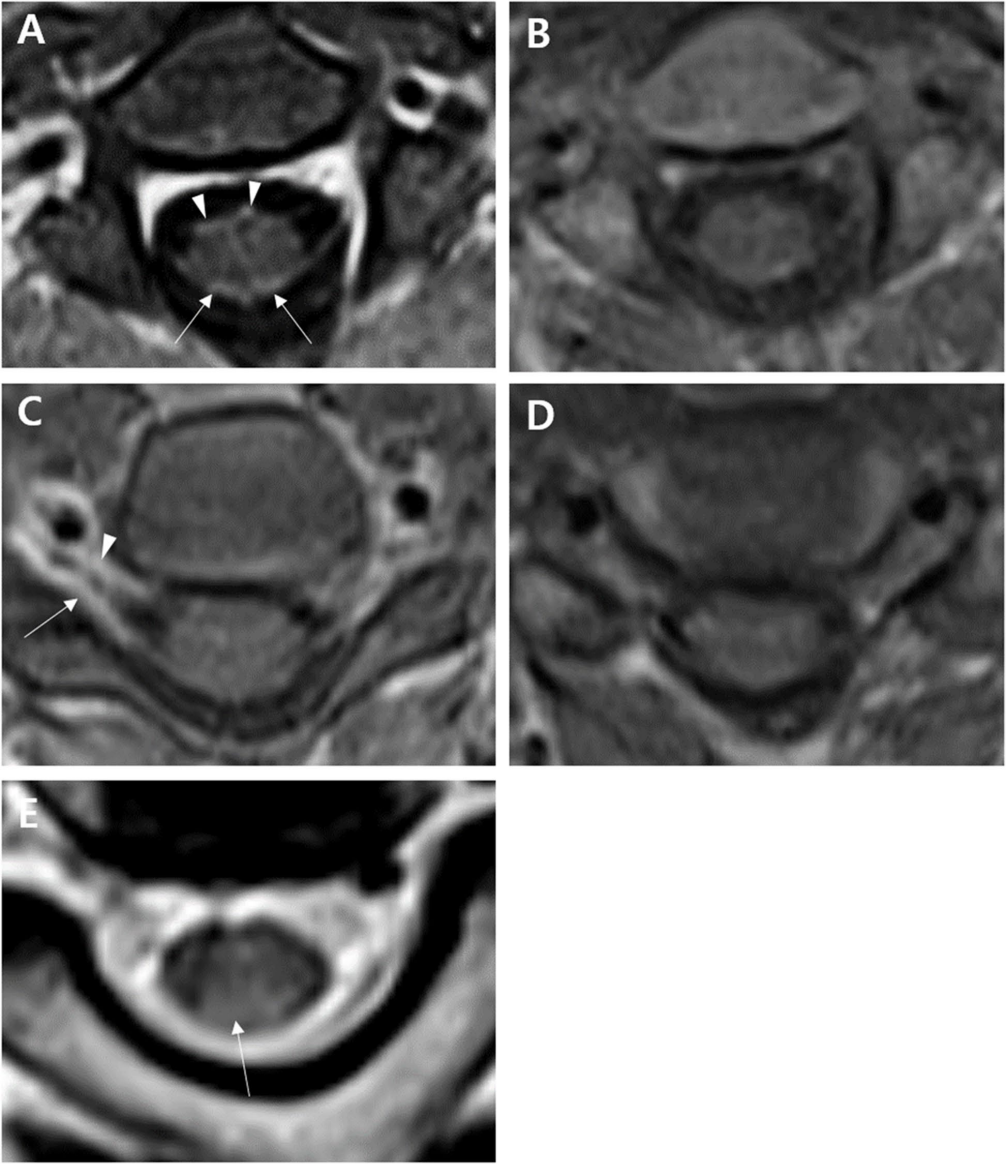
Cervical magnetic resonance imaging (MRI) taken at 18 days after the onset of acute autonomic and sensory neuropathy symptoms. Axial T1-gadolinum-enhanced MRI revealed enhancement (arrows) in cervical ventral (arrowhead) and dorsal (arrow) nerve roots at the level of C3 vertebral body (A) compared to non-enhanced image (B). At the level of C5-6 vertebra, contrast enhanced MRI also showed focal enhancement of right sixth cervical ventral (arrowhead) and dorsal nerve roots (arrow) (C and D). The T2-weighted MRI showed high signal intensity (arrow) in the posterior column of the cervical spinal cord at the C2-3 vertebral body level (E)
